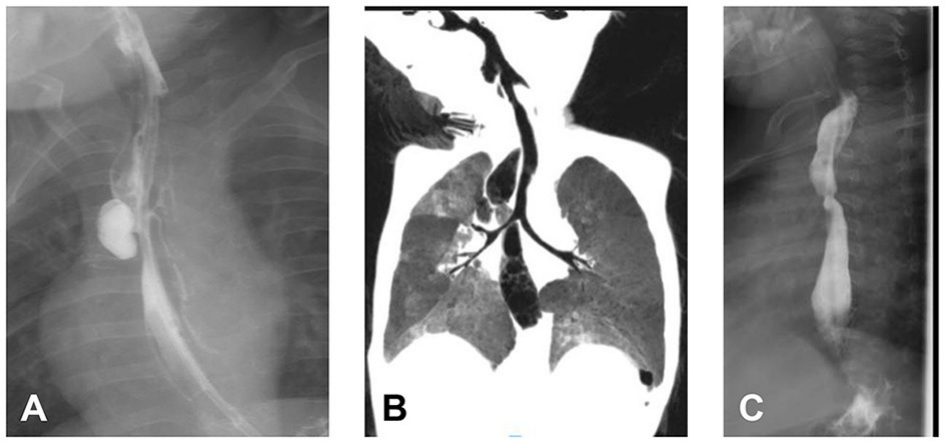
The pre-operative and postoperative radiography of patient 4. (A) Esophagogram revealed esophageal diverticulum (ED) (on the right side of the esophagus) combined with recurrent tracheoesophageal fistula (on the left side of the esophagus). (B) ED on chest computed tomography. (C) Two weeks after diverticulectomy.
